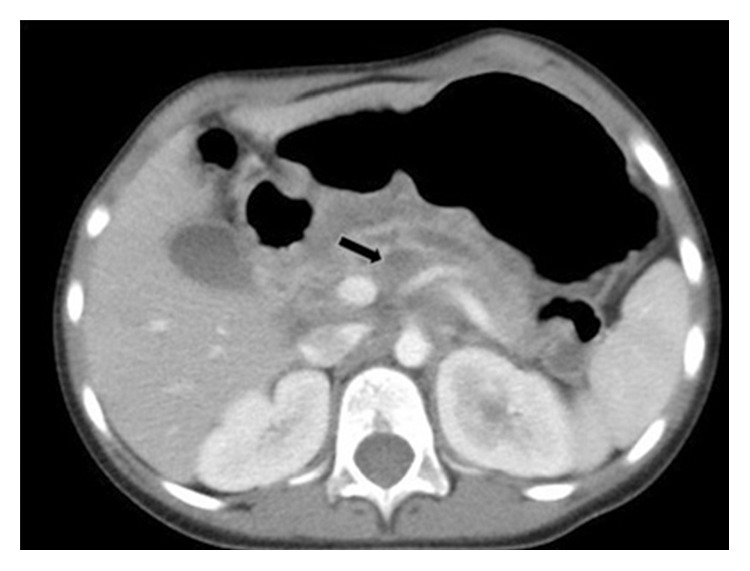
Abdominal enhanced CT showing a significant reduction in the cystic low-density shadow (arrow) of the head and neck of the pancreas one year after discharge.
